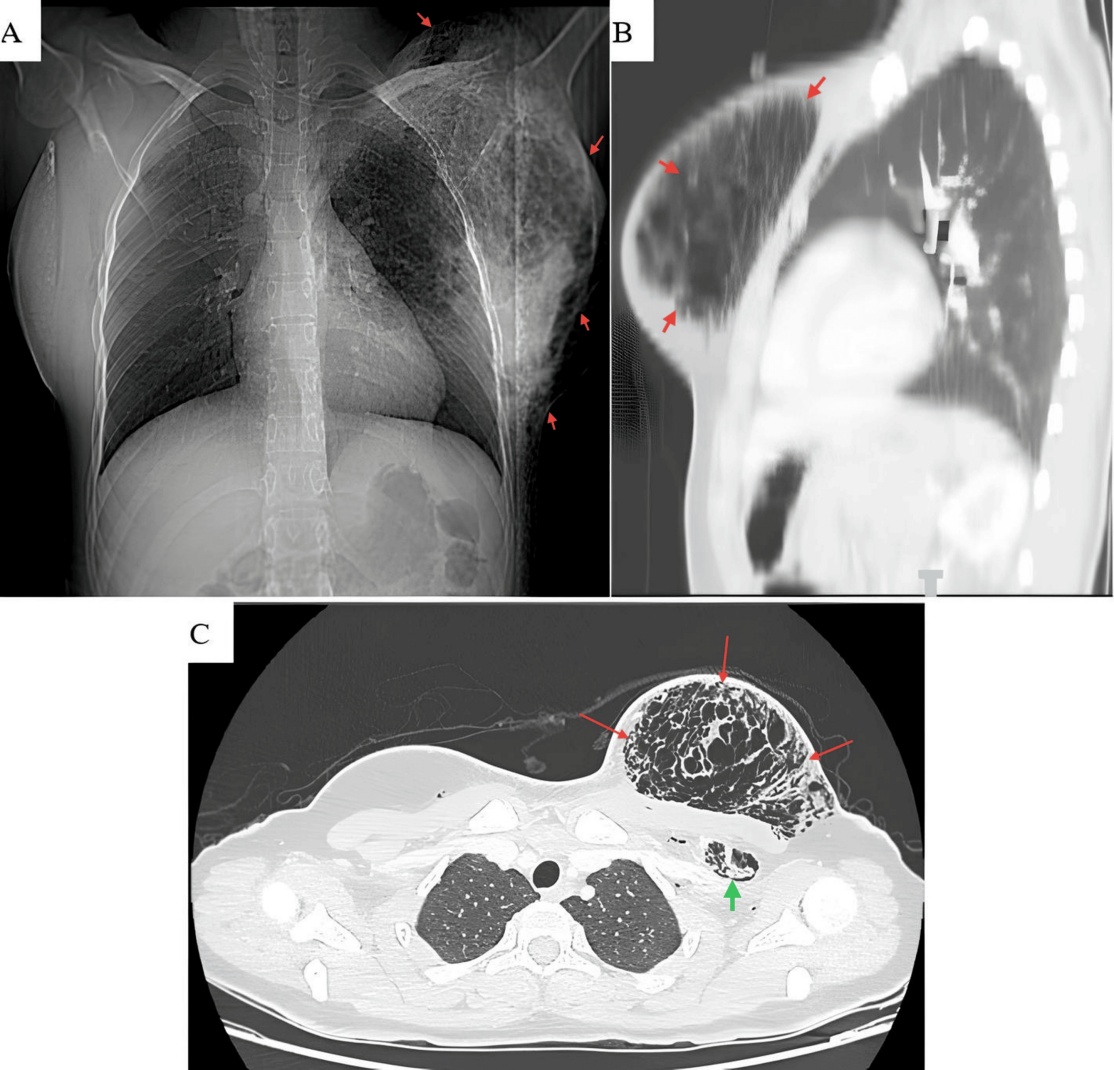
Imaging of left-sided pneumomastia (A) Posteroanterior chest X-ray revealing a large, well-circumscribed air collection along the left upper and lateral chest wall, replacing the majority of the left breast shadow (arrows), consistent with extrapulmonary air. (B) Sagittal contrast-enhanced chest computed tomography (lung window) showing a loculated air collection within the left breast (arrows). (C) Axial contrast-enhanced chest computed tomography (lung window) demonstrating an extensive, multiloculated air collection within the left breast, dissecting through the stromal connective tissue planes (red arrows). Air also tracks along the left anterior chest wall between the pectoralis major and minor muscles (green arrow). No evidence of pneumothorax or pneumomediastinum. No mediastinal or subcutaneous air tract is identified to suggest a broncho-cutaneous fistula.
